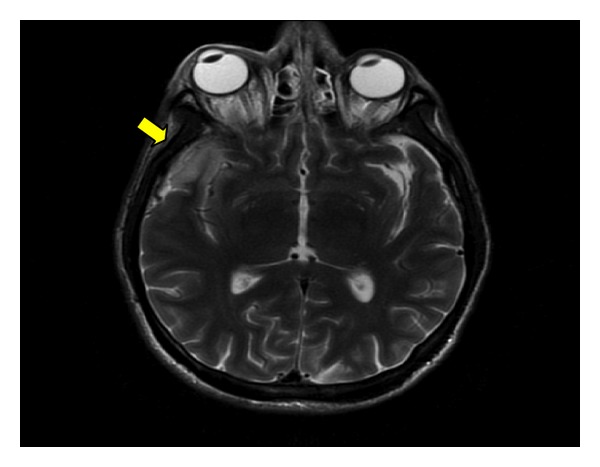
Head MRI T2w image showing hyperintensity in the right anterior temporal lobe.
